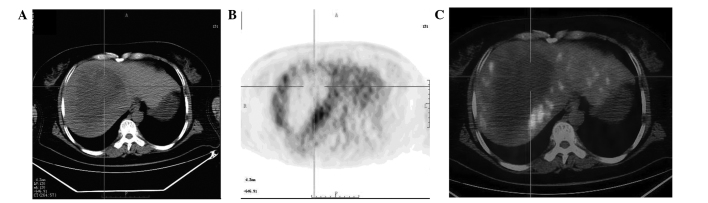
(A) Multiphasic CT images showing a large heterogeneous mass in the right lobe of the liver. (B) PET and (C) PET-CT images revealing an uneven ^18^F-fluorodeoxyglucose uptake shadow in the marginal portion of tumor, particularly within the upper edge of the tumor. The SUV_max_ was 10.3, while with the delayed scan the SUV_max_ was 13.2. CT, computed tomography; PET, positron emission tomography, SUV_max_, maximum standard uptake volume.
